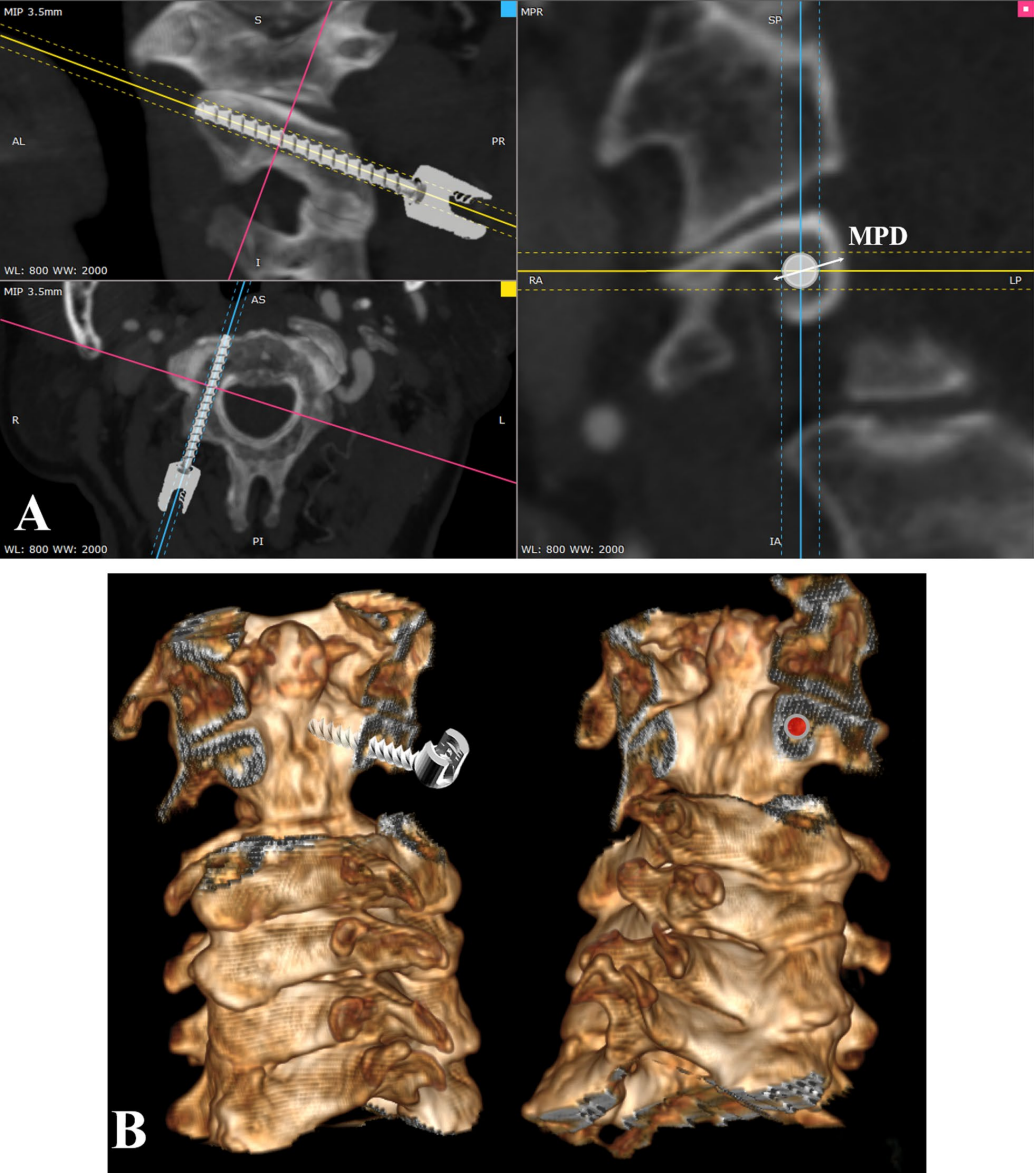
(A) Preoperative CTA multiplanar reconstruction was performed to measure MPD and evaluate the feasibility of C2 pedicle screw placement. (B) The measurement plane was shown on 3D reconstruction, which is perpendicular to the screw trajectory of both the axial and sagittal planes
